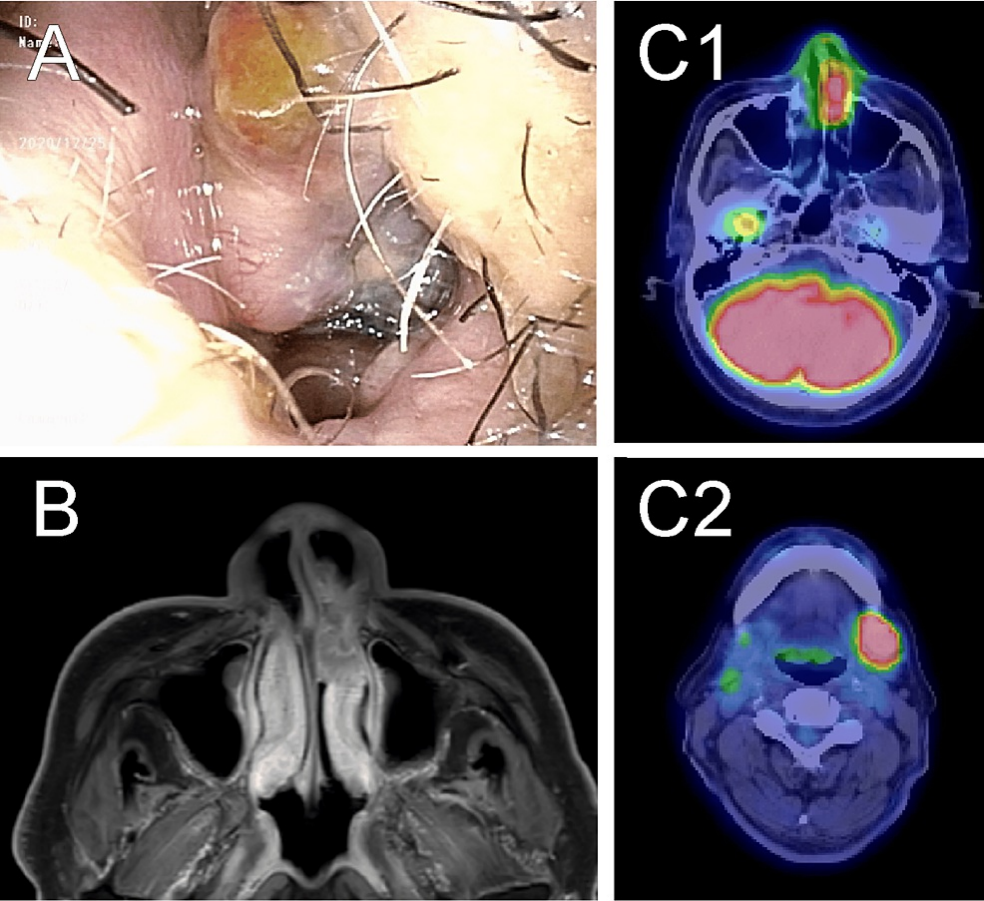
Case 3. A: Preoperative image of the tumor; B: Preoperative MRI showing a nasal cavity-occupying tumor; C: Positron emission tomography/CT images showing intense uptakes of 18F-FDG in the left nasal cavity (C1) and the left cervical lymph node (C2).
